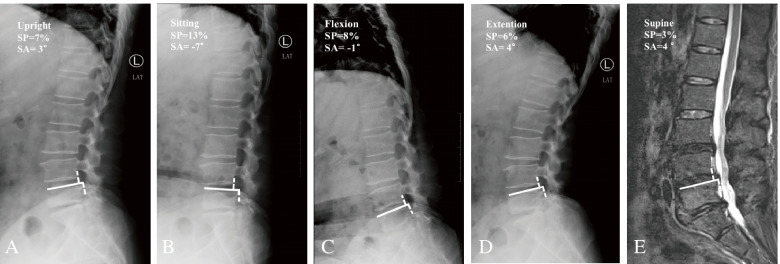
A 61-year-old female with L4/5 degenerative lumbar spondylolisthesis. The normal sitting radiograph showing a kyphotic slip angle at the involved segment B. The N-sMR analysis (B, E) demonstrated higher segmental mobility than FE (C, D) (10% vs. 2%)
